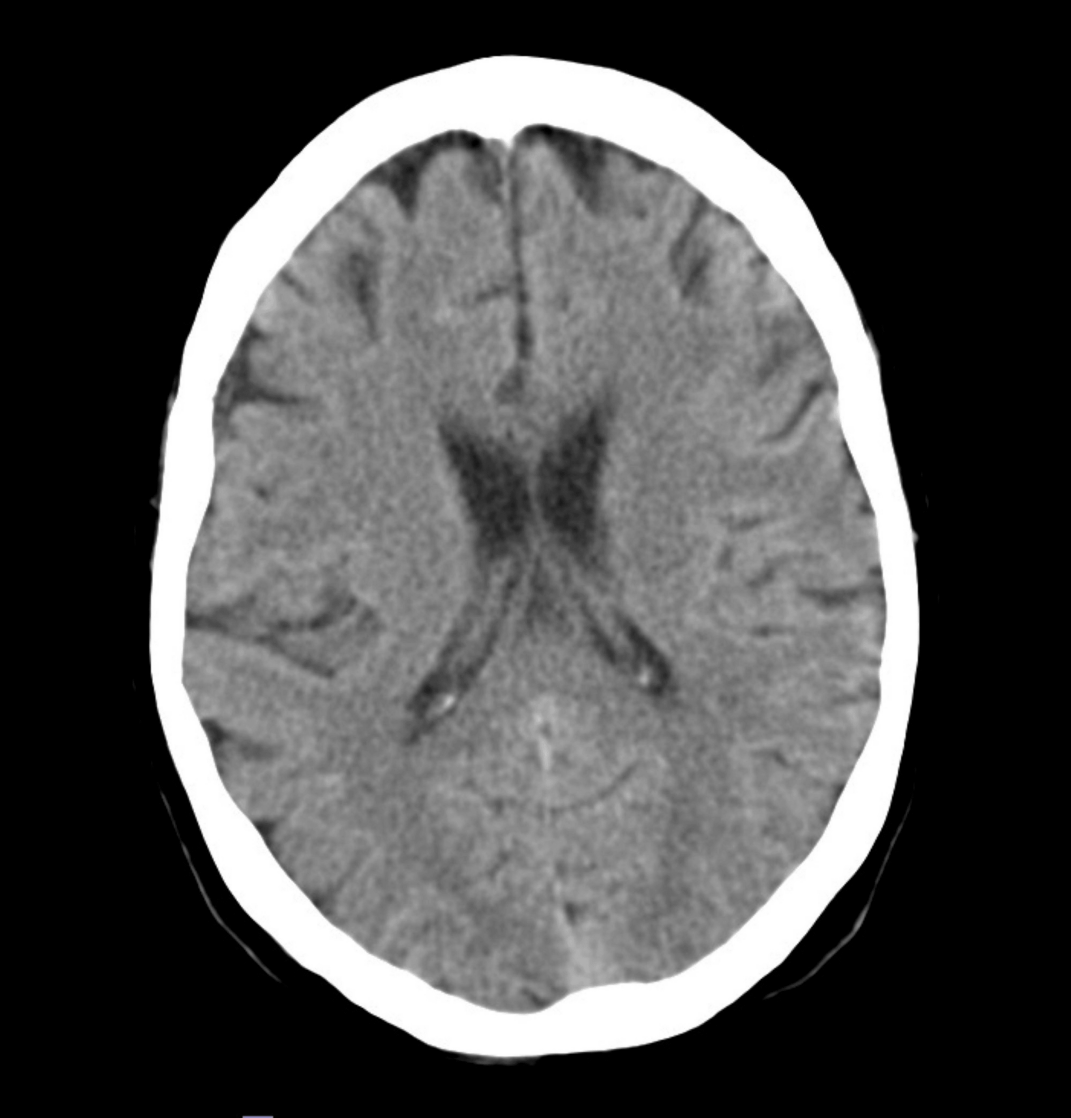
Head computed tomography without acute alterations
